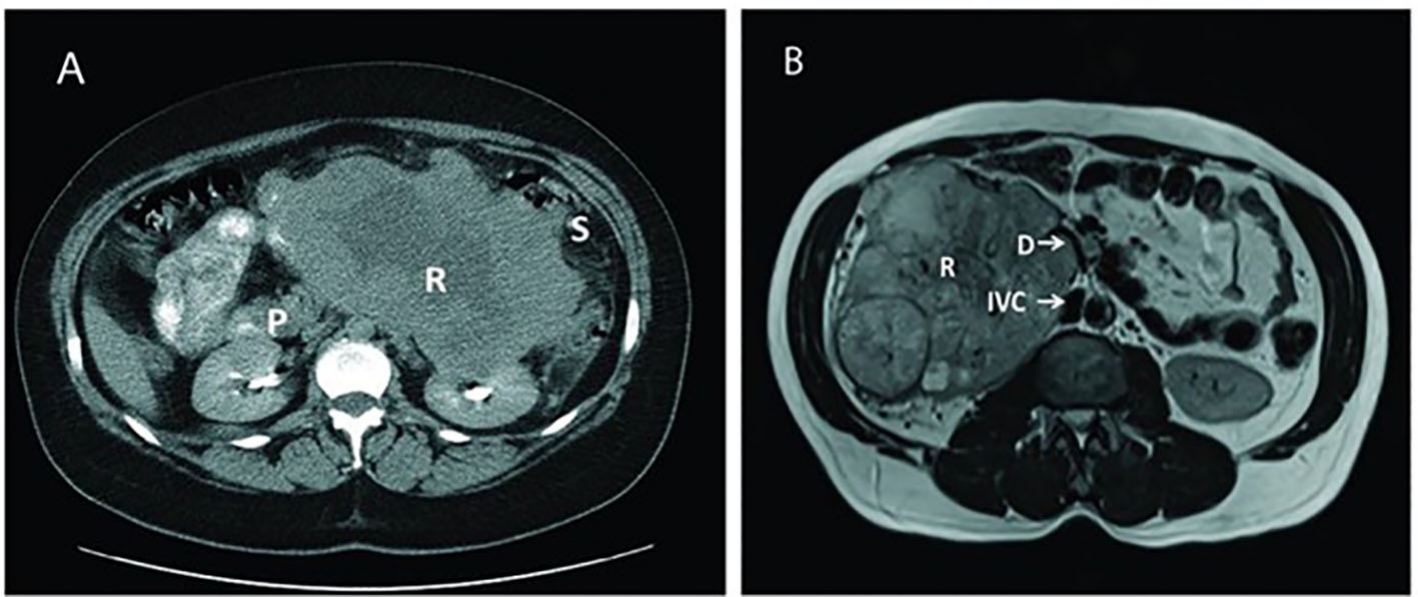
Computed tomography (CT) and magnetic resonance imaging (MRI) of two different patients with large renal mass. In the CT scan (A), the left kidney tumor shows the blurring of soft tissue boundaries around the pancreas, spleen, and splenic flexure, indicating it may have invaded the surrounding organs. Alternatively, imaging with MRI (B) confirms a large, mixed solid-cystic tumor on the right side of the kidney pushing on and narrowing the nearby duodenum. P: Pancreas; R: Renal Tumor; S: Splenic flexure of colon; IVC: Inferior Vena Cava: D: Duodenum (Reproduced from (42), under CC BY 4.0 license).
